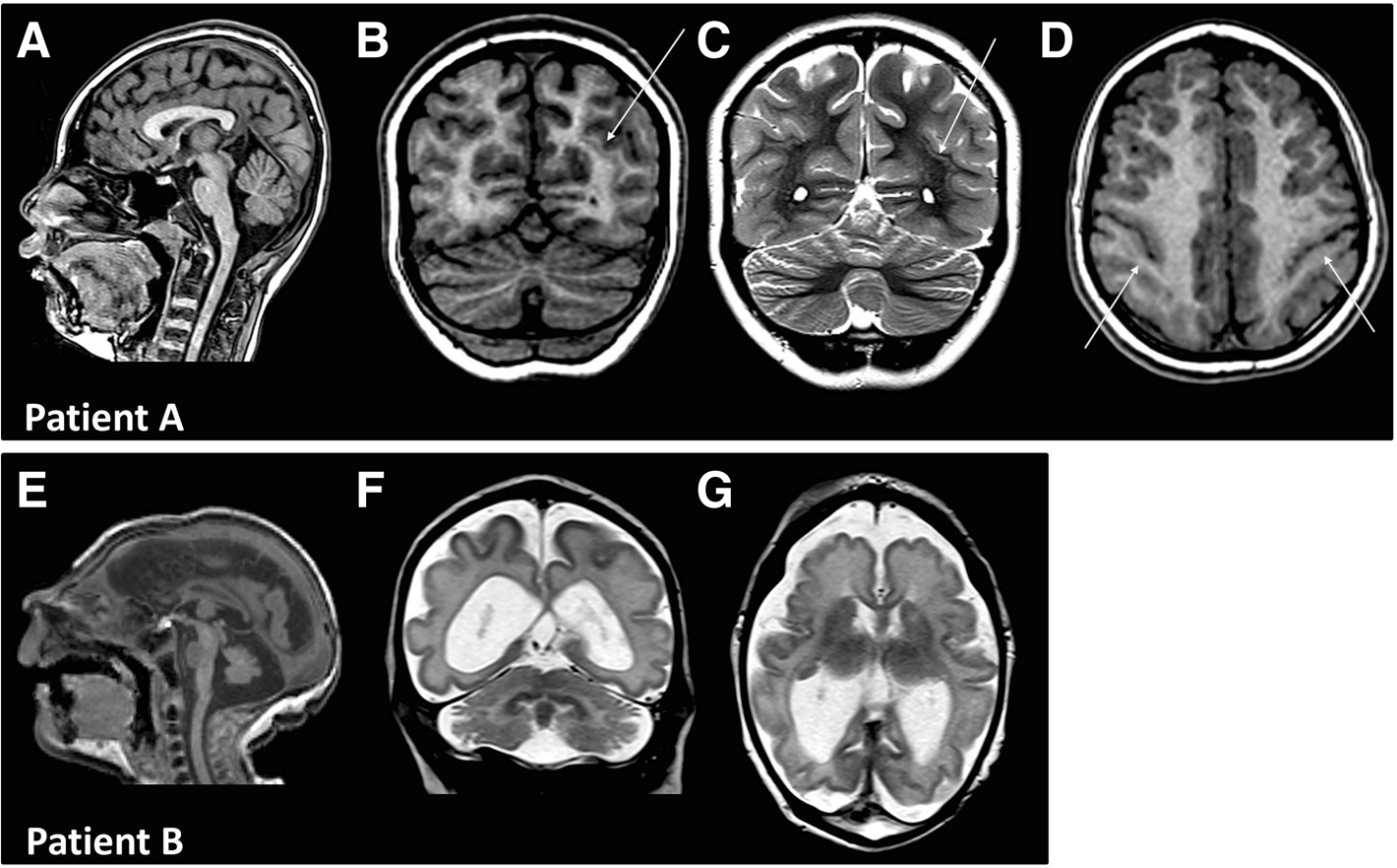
MRI Findings of Patient A with *TUBG1* p.Asp68Asn mutation and Patient B with *TUBG1* p.Arg341Trp mutation. a Sagittal T1-weighted MPRAGE image demonstrates reduced craniofacial ratio in this child with microcephaly and posteriorly predominant simplified cortical gyri. The cerebellar vermis is normal. The corpus callosum appears slightly thick. b and c Coronal (b) T1 weighted MPRAGE and (c) Coronal T2 weighted fast spin echo images demonstrating bilateral parietal band heterotopia, more pronounced on the left (arrow). d Axial T1 weighted MPRAGE image shows bilateral blurring of the grey-white interface along the central sulci, in keeping with focal cortical dysplasia (arrows). e Sagittal T1-weighted image demonstrates microcephaly, intact corpus callosum and normal pituitary. The pons and cerebellar vermis are small for gestational age, but are notably less affected than the supratentorial brain. f and g Coronal (f) and axial (g) T2-weighted images demonstrating dilated lateral ventricles and simplified gyral pattern. In (f) there is prominence of the cerebellar folia but normal-appearing dentate nuclei. In (g) the basal ganglia and thalami are small and poorly defined, with absent myelination of the posterior limb of internal capsule
